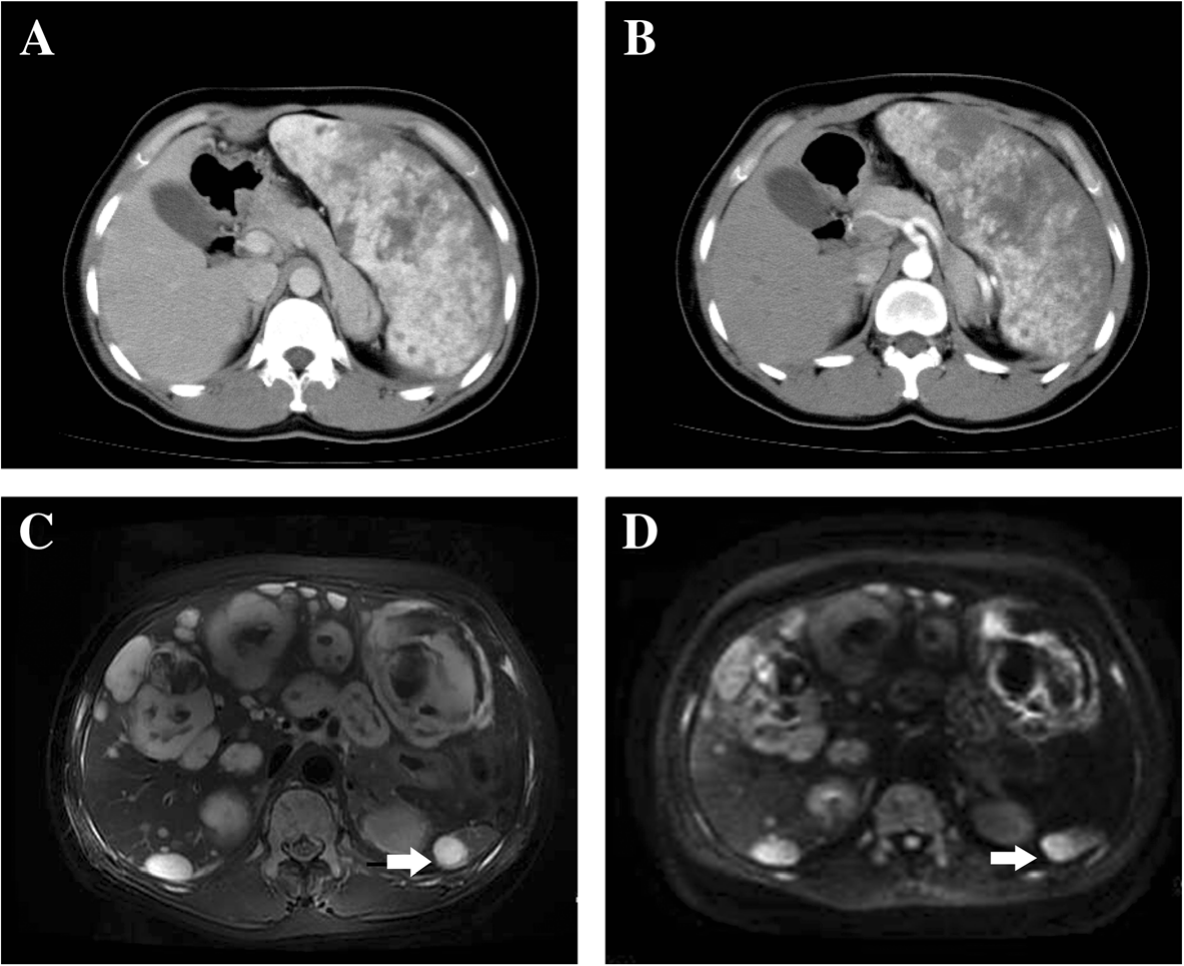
Abdomen CT scan in 2011 (a, b) demonstrated splenomegaly and multiple irregular low-density lesions. Liver, renal, adrenal, and pancreas are normal. The MRI in 2013 presented multiple cystic lesions in different sizes diffused in liver. The lesions displayed as inhomogeneous mixed signal, with high signal intensity on T2-weighted image (T2WI, c) and diffusion-weighted image (DWI, d). Some lesions that indicated eccentric or central low signal intensity were suspected intertumoral hemorrhage. Nodule lesion with abnormal sign as that in the liver was also found in remnant accessory spleen (*arrow*)
